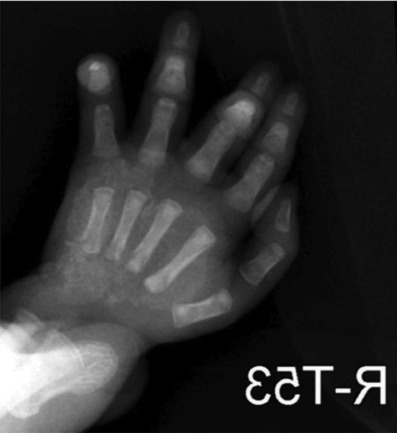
X-ray of the Right hand with medial rotation.
